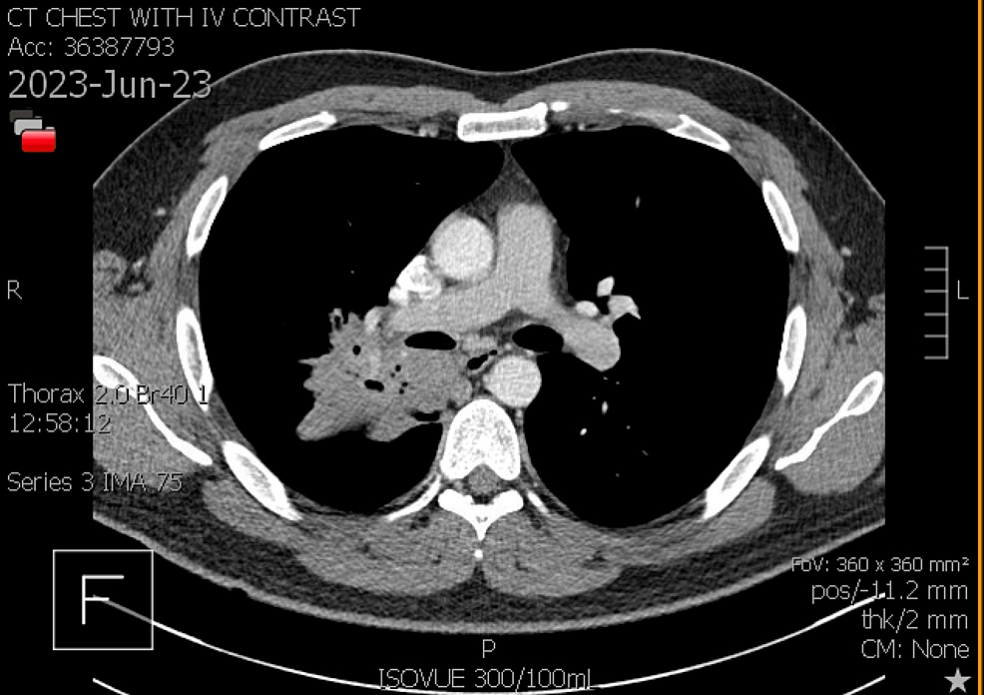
CT chest with IV contrast clearly showing a mass in the right upper lobe posterior segment (axial view)
